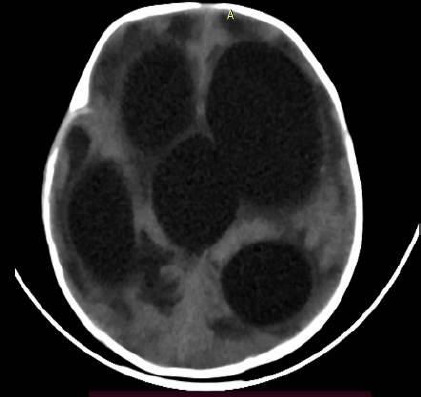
CT of the brain showing dilated ventricles.
